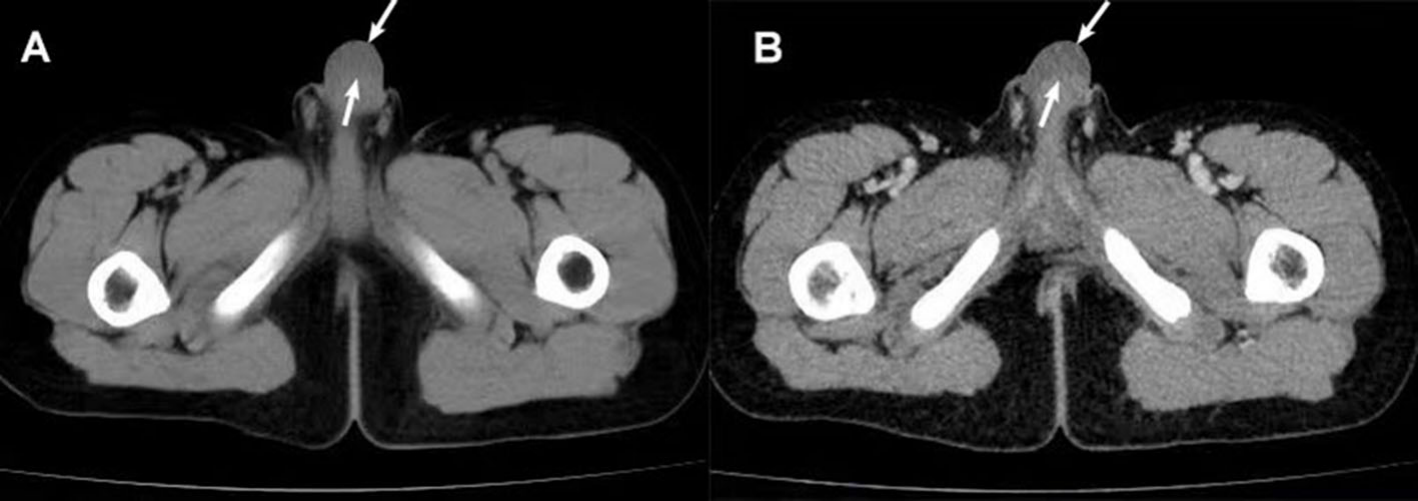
CT scan findings of the penile mass. (A) CT plain scan: a uniform low-density mass (arrow) was seen under the penile skin. (B) Enhanced CT: No definite enhancement of the mass (arrow) after enhancement.
